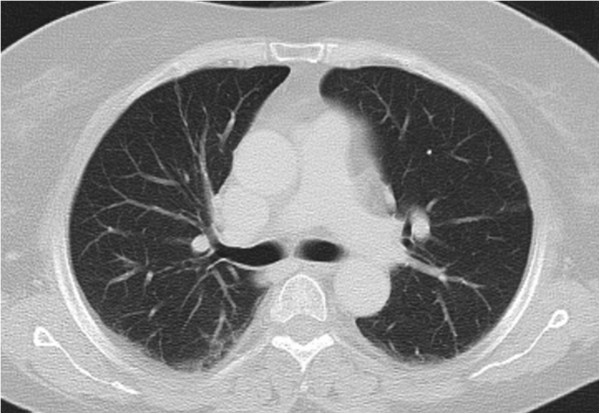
Restaging with CT three months after the adrenalectomy showed disappearance of all KS-induced pulmonary nodules.
